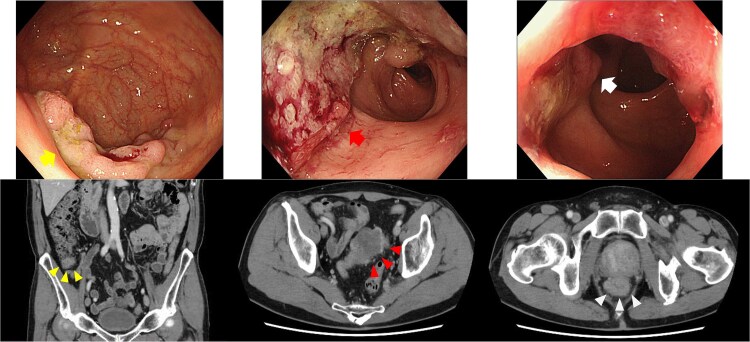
Endoscopy and enhanced CT endoscopy showed tumors in the cecum (LST-G, upper left), sigmoid (type 2, upper middle), and rectum (type 2, upper right). Enhanced CT provided important insights into the wall thickness in the cecum (lower left), sigmoid colon (lower middle), and rectum (lower right).
